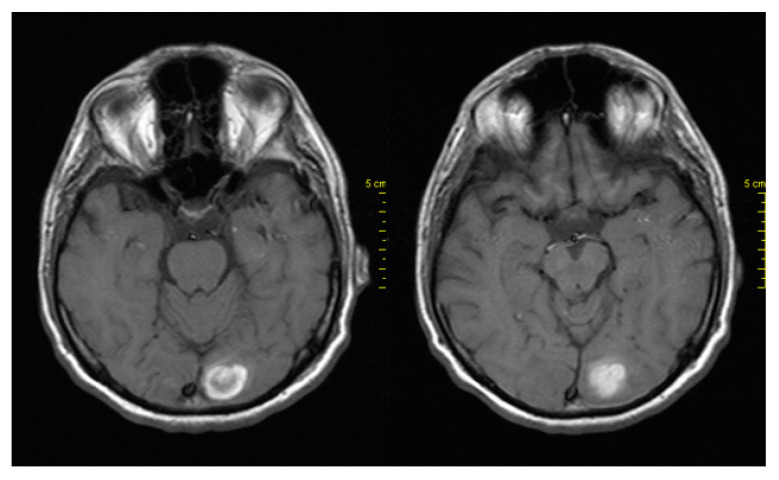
MRI showing brain metastases in the left occipital lobe.
